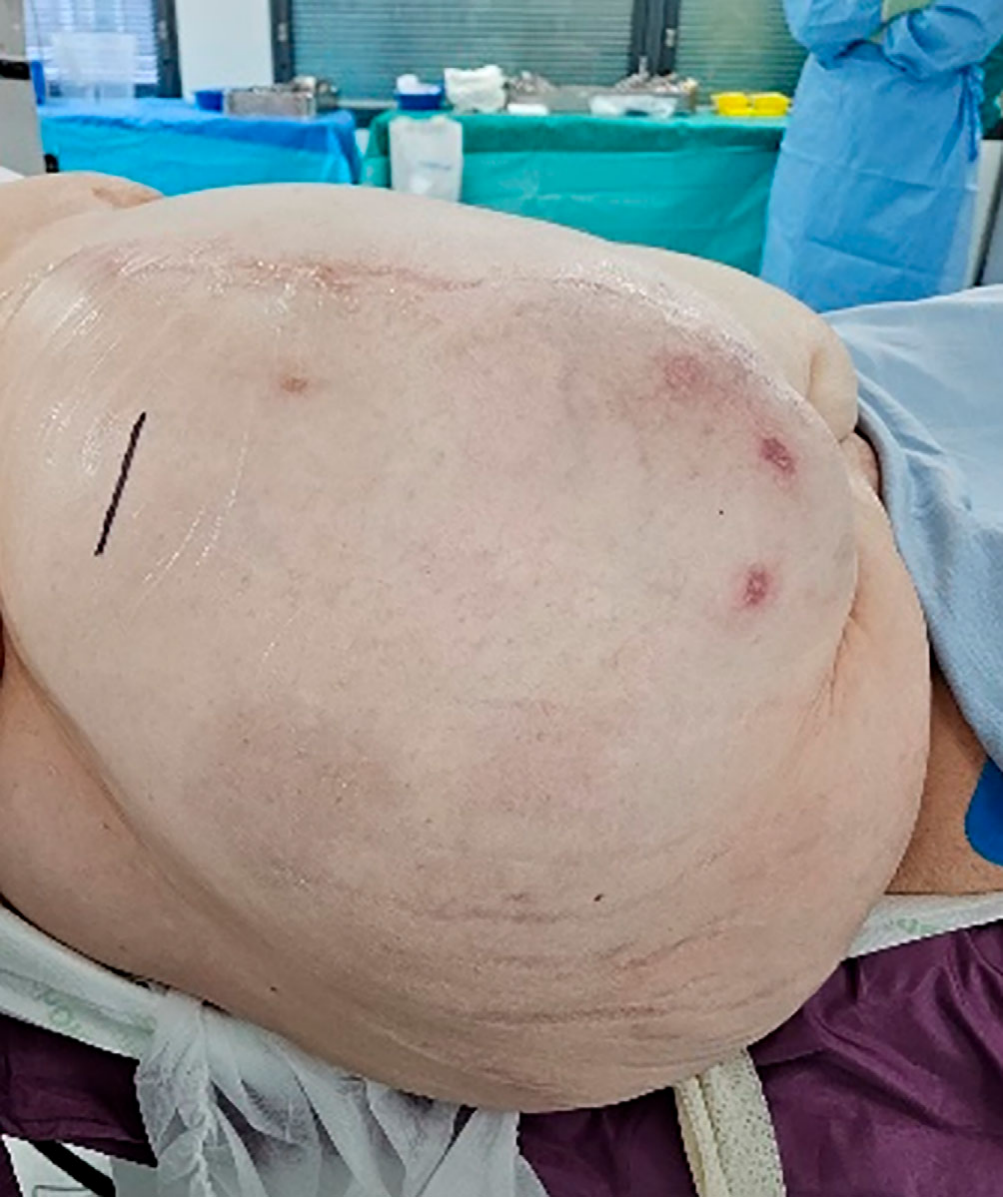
Ultrasound guided confirmation of the gallbladder in the hernia sac, prior to the transverse incision.
